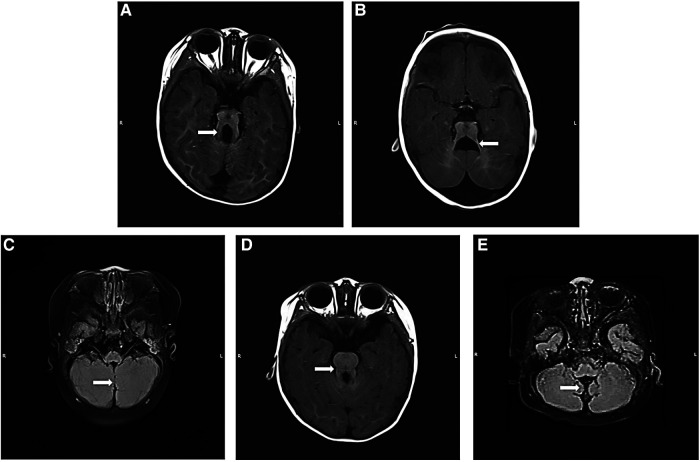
Brain MRI findings of Joubert syndrome children. (A) Axial T1WI: “molar tooth sign”; (B) axial T1WI: “bat wing sign”; (C) T2WI flair: “midline fissure sign”; (D) axial T1WI: the typical imaging findings of a patient with *CPLANE1* variant Joubert syndrome showing a “molar tooth sign”; this sign was less obvious in other patients; (E) T2WI flair: typical “hypoplasia of the cerebellar vermis”, “morphological abnormality of the fourth ventricle” and “thickened, elongated superior cerebellar peduncles” in the child with *CHD7* gene variant. T1WI, T1 weighted imaging; T2WI, T2 weighted imaging.
